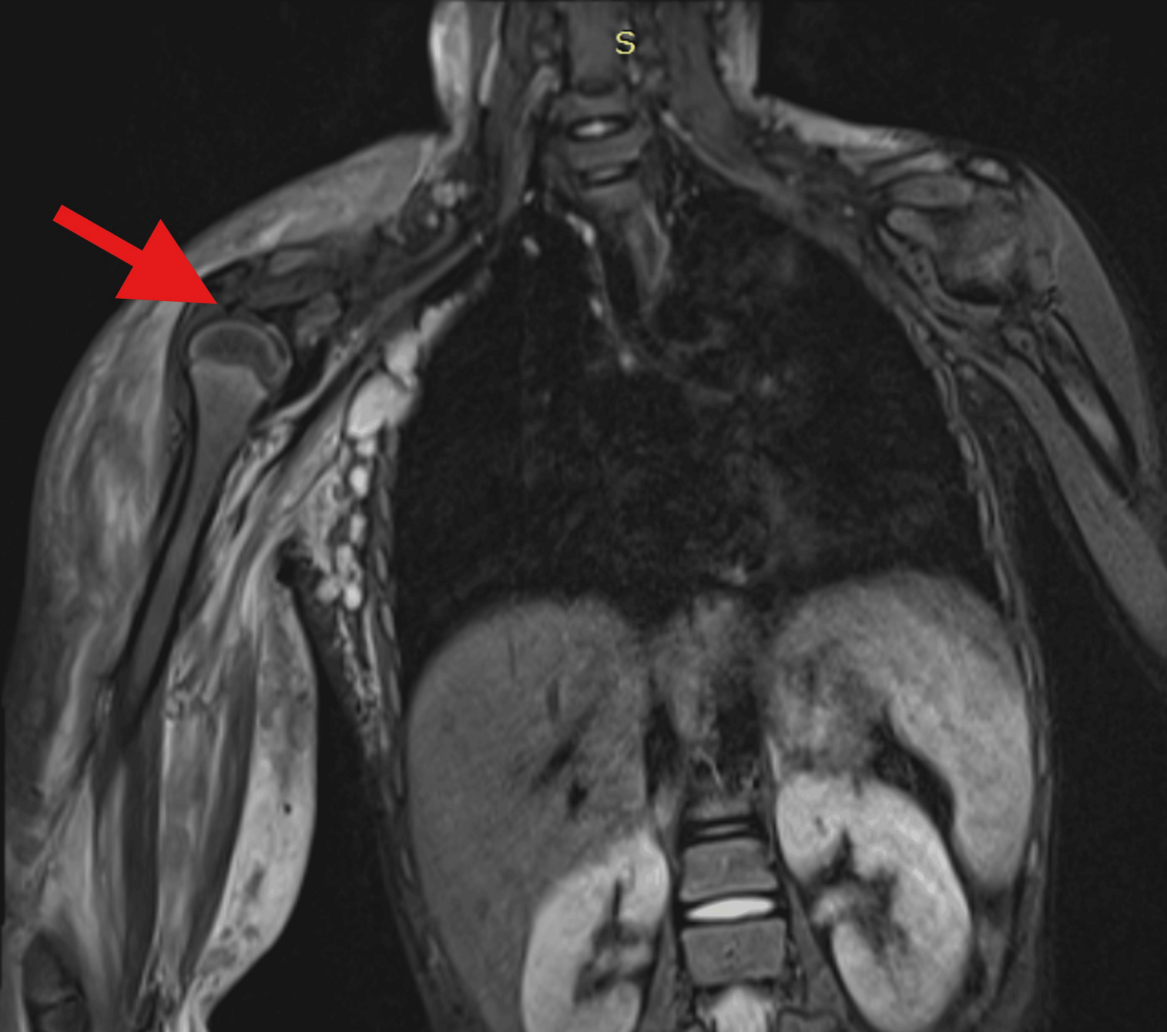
T2-STIR sequence revealing hyperintense edema in intermuscular and subcutaneous planes with intramuscular edema and enlarged axillary and pectoral lymph nodes T2-STIR: T2-weighted short-tau inversion recovery
